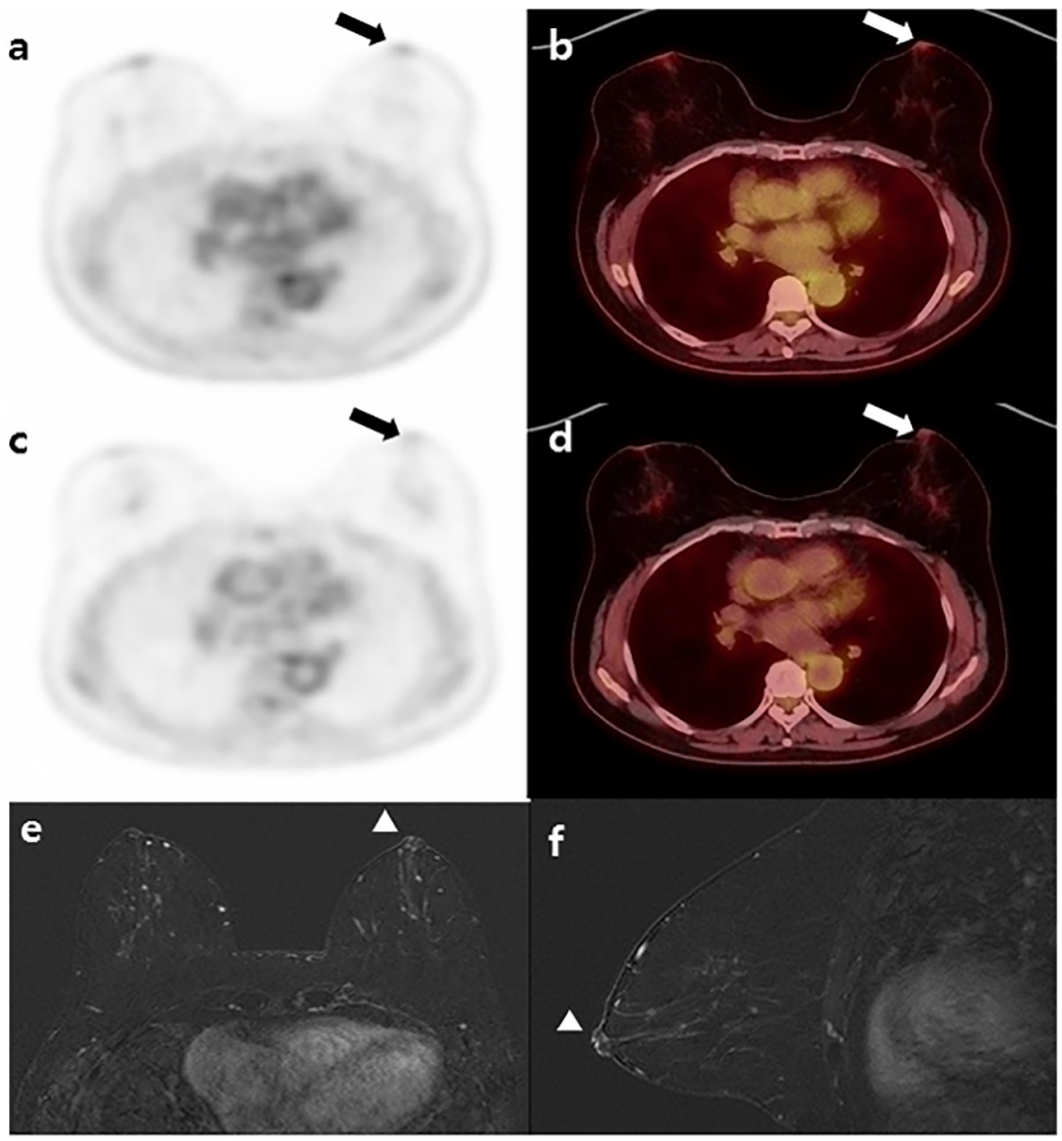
A 48-year-old patient with IDC in the left breast: (a, b) Early phase ^18^F-FDG PET and fusion PET/CT; (c,d) Delayed phase PET and fusion PET/CT show no significant FDG uptake in the NAC of the left breast (arrow), NAC-SUVR_early_ = 0.95; NAC-SUVR_delay_ = 0.92; (e) Contrast-enhanced MRI with axial view and (f) MRI with sagittal view; MRI reveals no definite evidence of abnormal contrast enhancement of the NAC in the left breast (arrowhead). This patient underwent breast conserving surgery, and pathologic NAC non-involvement was confirmed.
